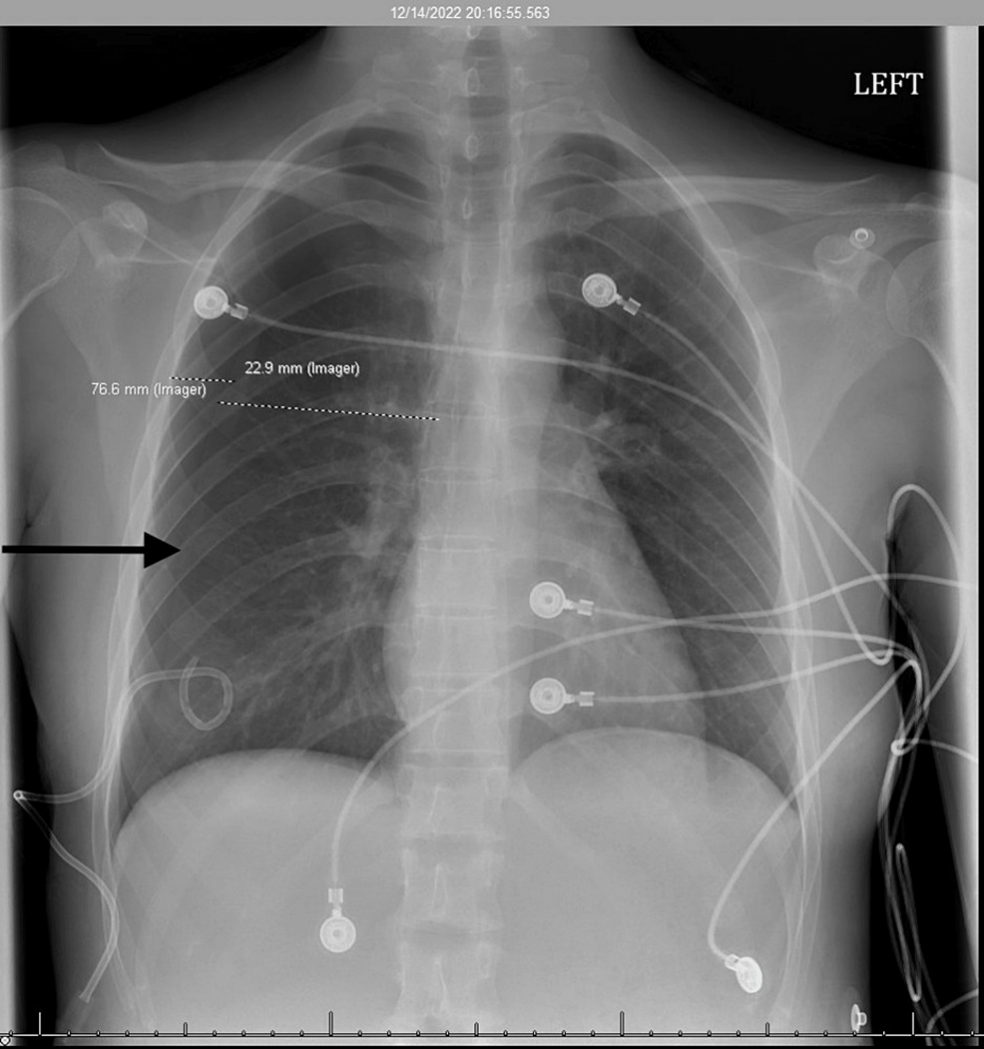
Chest x-ray #3 Impression: No consolidation, mass or edema. No pleural effusion. There is interval decrease in the size of the right pneumothorax measured approximately 40%.
